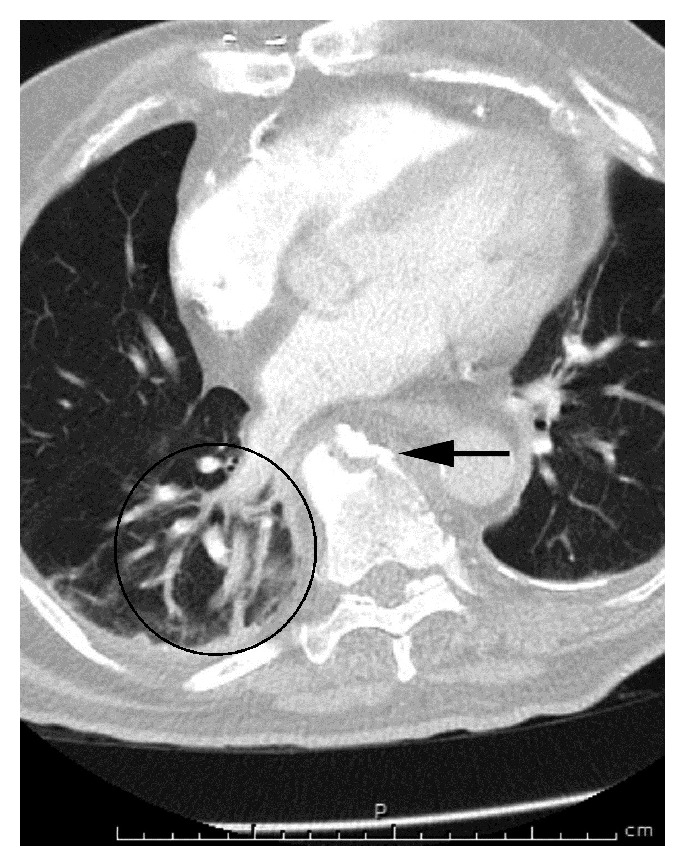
Axial CT angiogram of the chest showing a T8 vertebral fracture (*arrow*) and lung hemorrhage or possible contusion in the right lung base (*black circle*).
